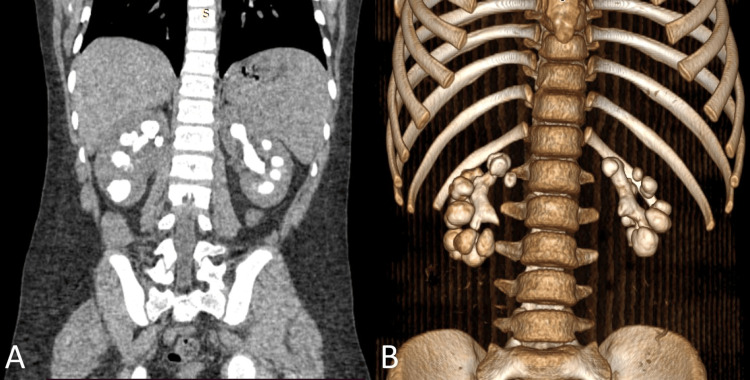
A) CT scan of the coronal section; B) 3D reconstruction of a CT scan, anterior view
